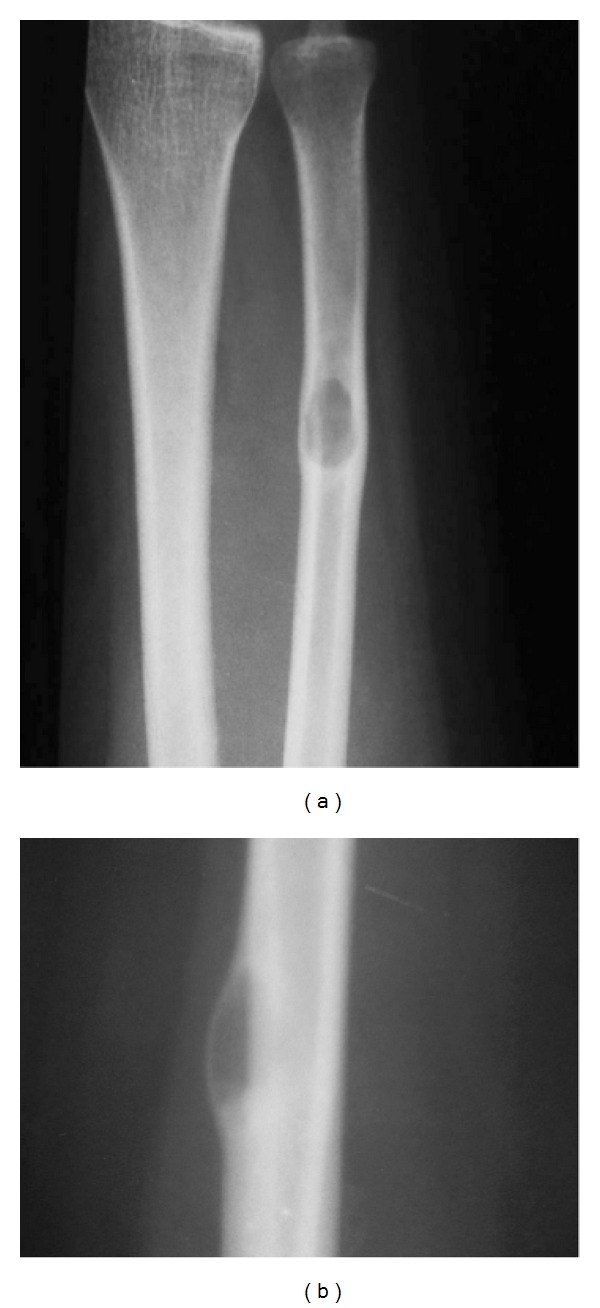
(a) antroposterior radiography revealed osteolytic well-defined lesion without marginal sclerosis and cortical destruction. (b) lateral radiography revealed eccentric exophytically osteolytic lesion without calcification or marginal sclerosis.
